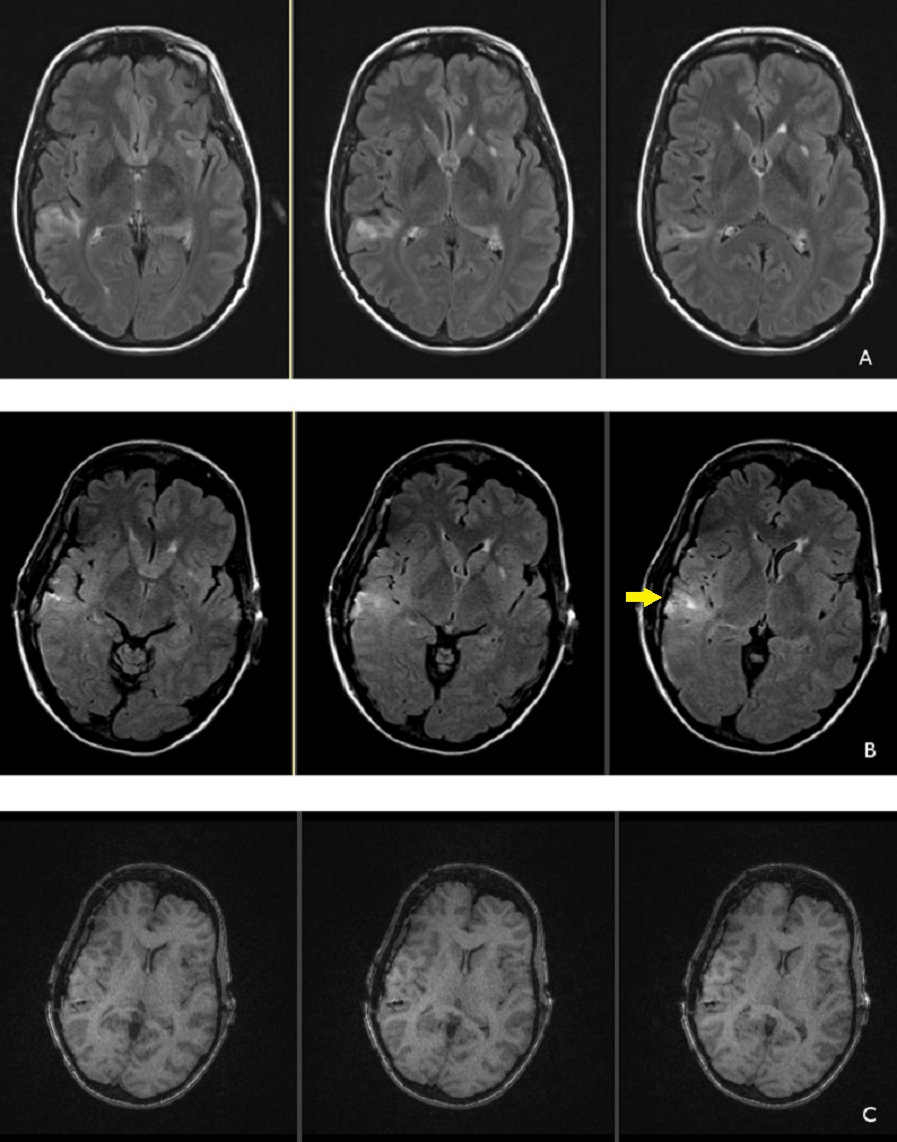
Magnetic resonance images demonstrating postoperative changes Preoperative MRI (A) demonstrating fluid attenuated inversion recovery (FLAIR) hyperintensity in the right posterior parietal area. Postoperative MRI (B) showing similar hyperintensity after grid placement with subtle increase in FLAIR hyperintensity related to grid artifact (arrow). No evidence of cortical contusion is noted. Postoperative changes, T1 non-contrast sequences (C) showing absence of postoperative intraparenchymal hemorrhage.
